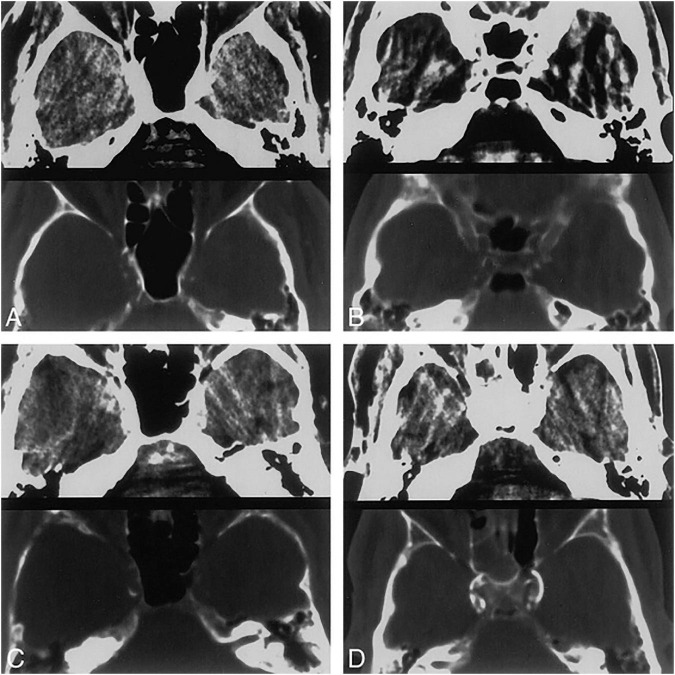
Four different CT patterns of calcification. 5-mm-thick axial CT scans at the level of the carotid siphon. Brain (top) and bone (bottom) windows are shown in each image. (A) Thin, discontinuous calcification, bilaterally. (B) Thin, continuous calcification, right side. (C) Thick, discontinuous calcification, right side. (D) Thick, continuous calcification, bilaterally. Republished with permission of American Society of Neuroradiology, from Woodcock et al. (1999); permission conveyed through Copyright Clearance Center, Inc.
